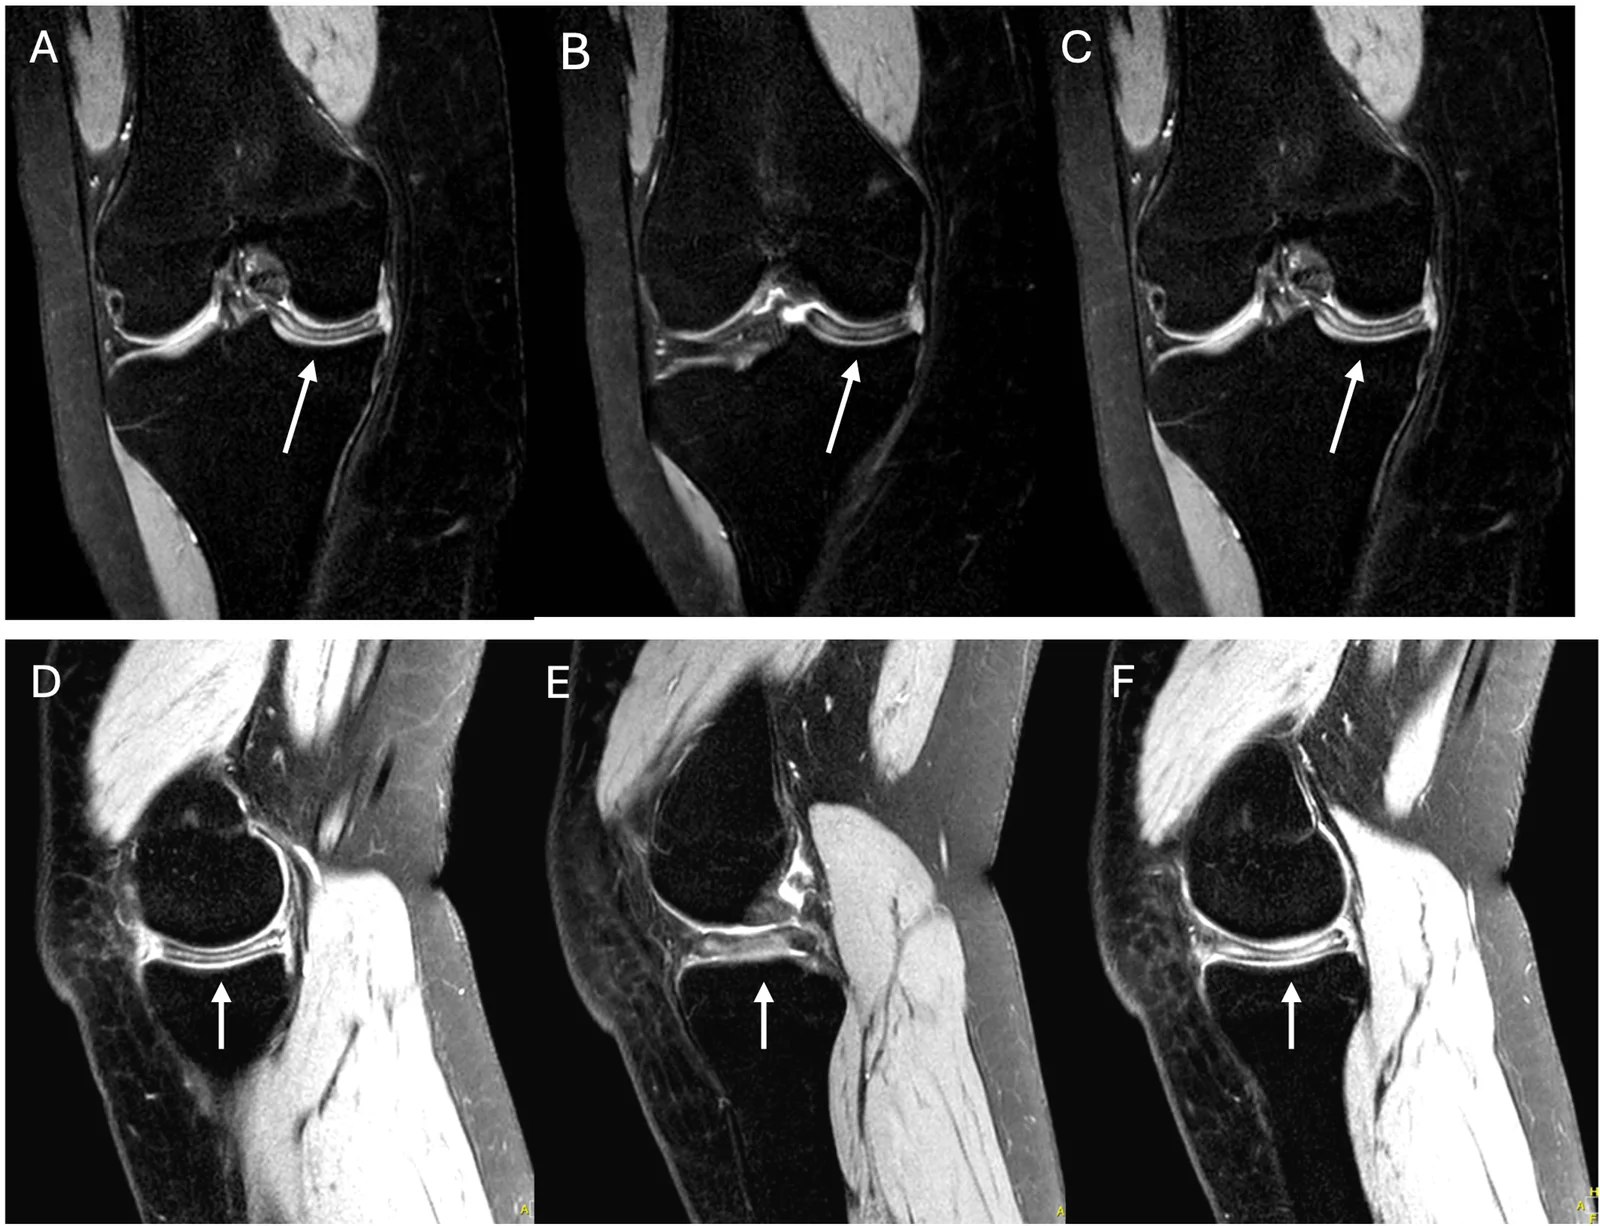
Preoperative MRI demonstrating a discoid medial meniscus. (A-C) Right knee coronal fluid-sensitive MRI sequences progressing from anterior (A) to posterior (C), confirming a complete discoid medial meniscus. A consistent slab-like width exceeding 5 mm forms a complete, continuous tissue bridge extending from the peripheral capsule to the intercondylar notch, thereby covering >80% of the medial tibial plateau (white arrows). This morphology persists without tapering across all three consecutive slices, satisfying the multislice diagnostic criteria. Linear high-signal-intensity fluid tracks transversely through the central body of the discoid meniscus. The horizontal tear originates near the central free edge of the discoid “slab” (C) and propagates peripherally along the horizontal midplane, completely dividing the body of the meniscus into superior and inferior laminae. The lateral meniscus demonstrates normal triangular morphology (white asterisk, A). (D-F) Right knee sagittal fluid-sensitive MRI sequences progressing from lateral (D) to medial (F). These images demonstrate classic features of a complete discoid medial meniscus (white arrows). The sequence reveals abnormal, continuous meniscal tissue spanning three consecutive slices, satisfying the sagittal diagnostic criterion of a continuous “bow tie” sign across three slices. Instead of transitioning normally from the peripheral body into distinct anterior and posterior triangular horns, the meniscal tissue remains thickened, block-like, and continuous throughout the joint compartment, confirming complete discoid morphology.
